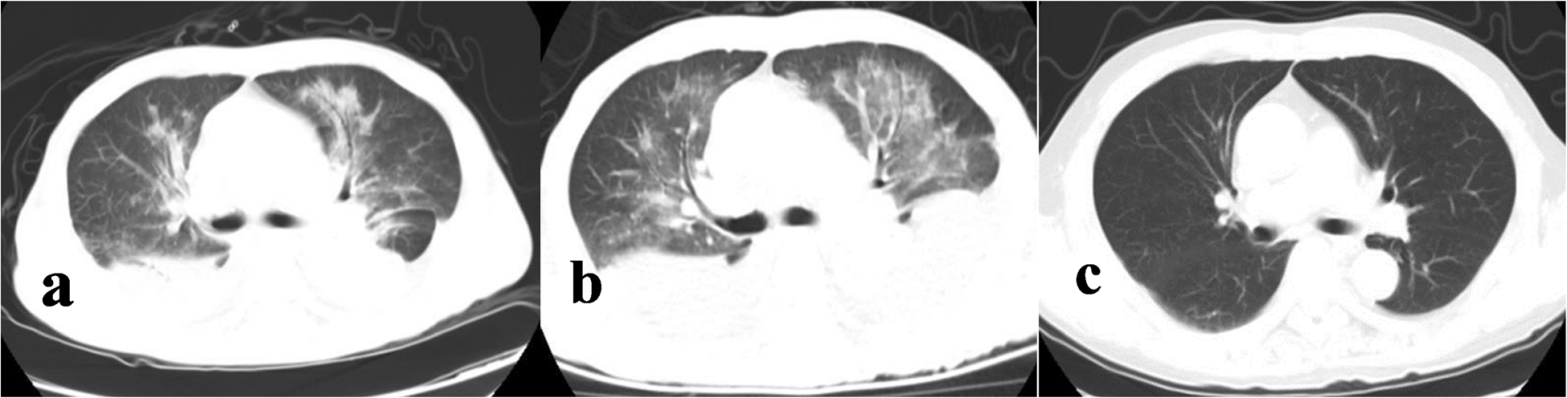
Characteristics of Chest CT during treatment. Chest CT showed infiltrates on both lungs on admission (a) and the infiltrates increased on day 7 after adjusting antibiotics treatment (b). Four weeks after starting linezolid therapy, the infiltrates disappeared (c)
